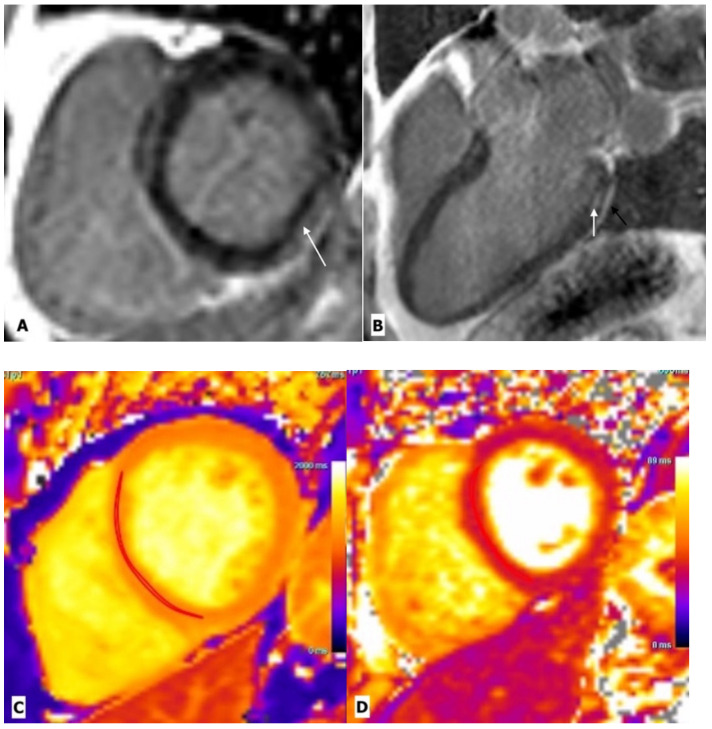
Cardiac magnetic resonance imaging in a 43-year-old man with HIV-related perimyocarditis. (A) Mid-ventricular short-axis and (B) 3-chamber long-axis images showing linear mid-wall enhancement in the basal inferior and inferolateral lateral walls (white arrows). The overlying pericardium also shows mild thickening and enhancement. (C) T1 map and (D) T2 map at the mid-LV show a markedly elevated myocardial T1 time of 1214 ms (normal 1104 ms at 3.0 Tesla) and T2 time of 44 ms (normal 37.4 ms at 3.0 Tesla) consistent with diffuse myocardial fibrosis.
